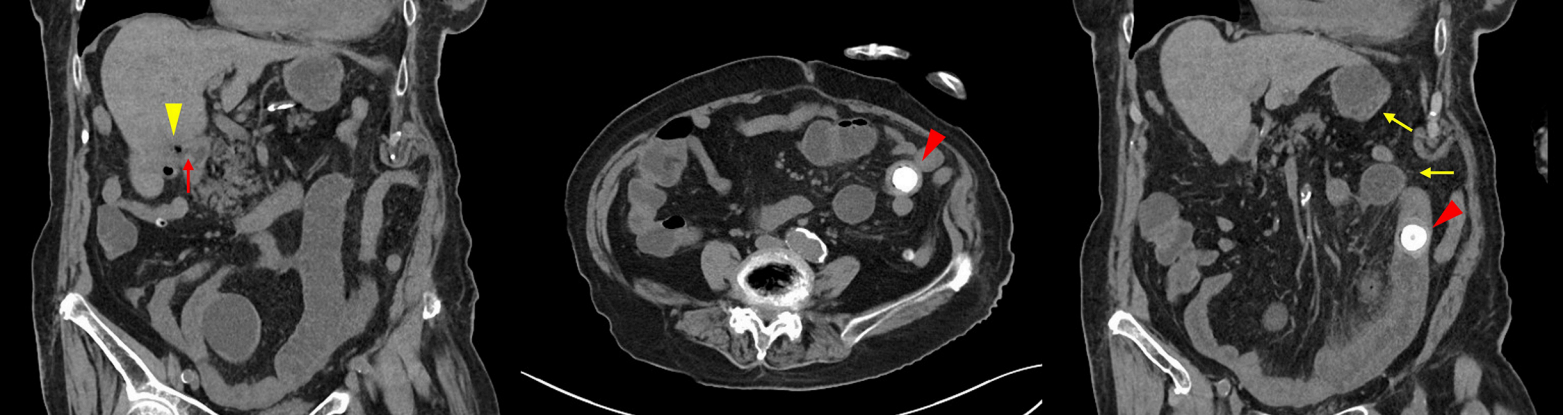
Abdominal computed tomography scans on the present visit showing pneumobilia (yellow arrowhead), the cholecystoduodenal fistula (red arrow), a gallstone (red arrowheads) in the small intestine, and small-bowel obstruction (yellow arrows).
